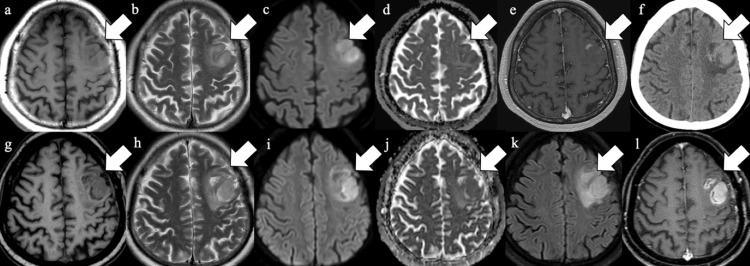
Imaging features The tumor showed slight hypo-intensity on MRI T1-weighted images (white arrow) (a), hyperintensity on T2-weighted images (white arrow) (b), iso- to hyperintensity on diffusion-weighted images (white arrow) (c), and iso- to hyperintensity on apparent diffusion coefficient images (white arrow) (d). Part of the tumor showed homogeneous enhancement after contrast administration (white arrow) (e). CT and MRI evaluated at our hospital (white arrows) (f-l). Brain CT revealed a high-density lesion in the left frontal lobe (white arrow) (f). The tumor showed slight hypointensity on T1-weighted images (white arrow) (g), hyperintensity on T2-weighted images (white arrow) (h), hyperintensity on fluid-attenuated inversion recovery images (white arrow) (i), iso- to hyperintensity on diffusion-weighted images (white arrow) (j), and iso- to hyperintensity on apparent diffusion coefficient images (white arrow) (k). The tumor showed heterogeneous enhancement after contrast administration (white arrow) (l).
